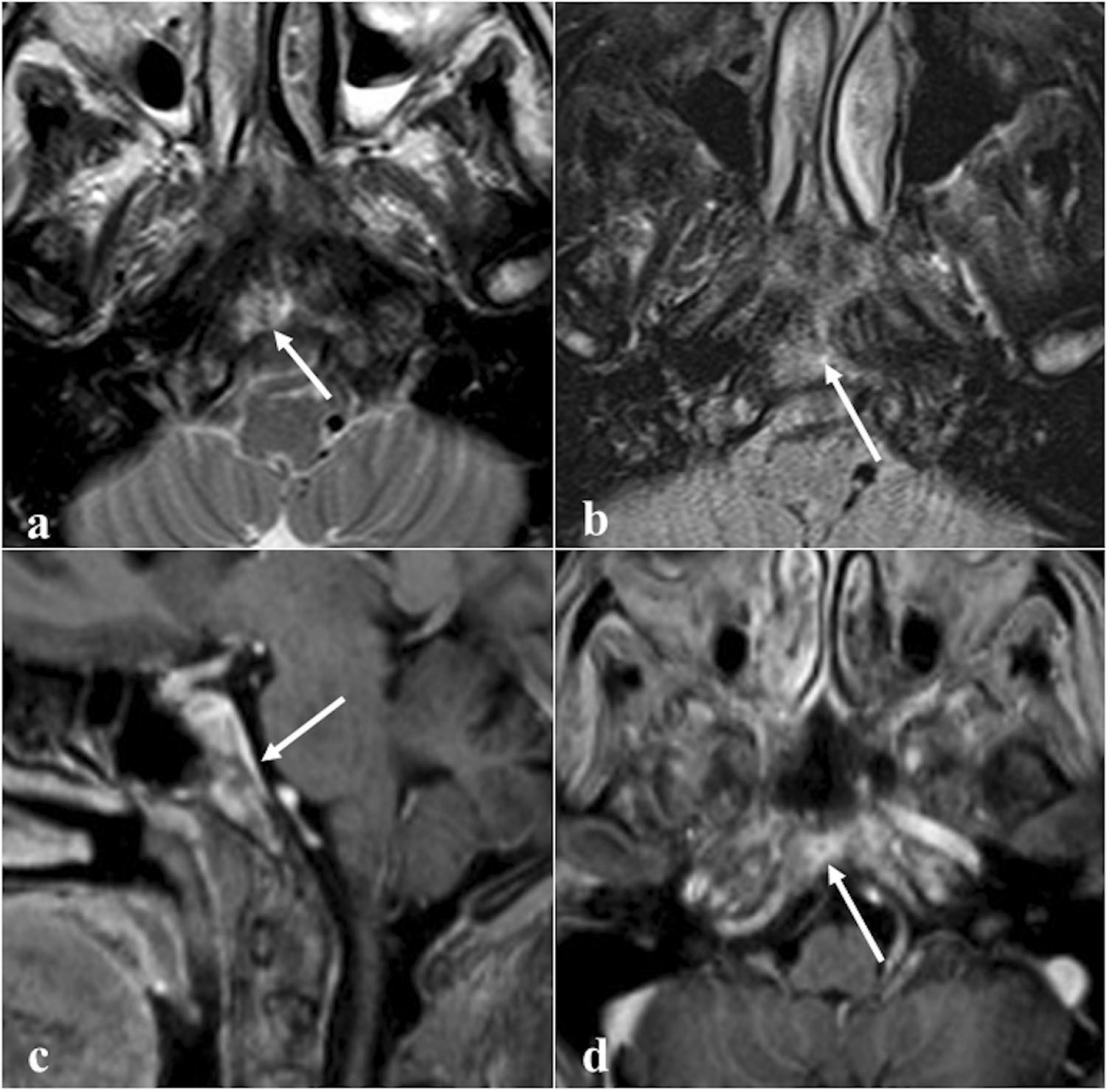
Clival metastasis in a 58-year-old female patient with known breast cancer and focal retro-orbital uptake on scintigraphy (not shown here). Axial T2W (a), axial fluid attenuation inversion recovery (b), sagittal T1W (c), and axial T1W fat-saturated CE (d) MRI images show a hypervascular clival metastasis (white arrows)
